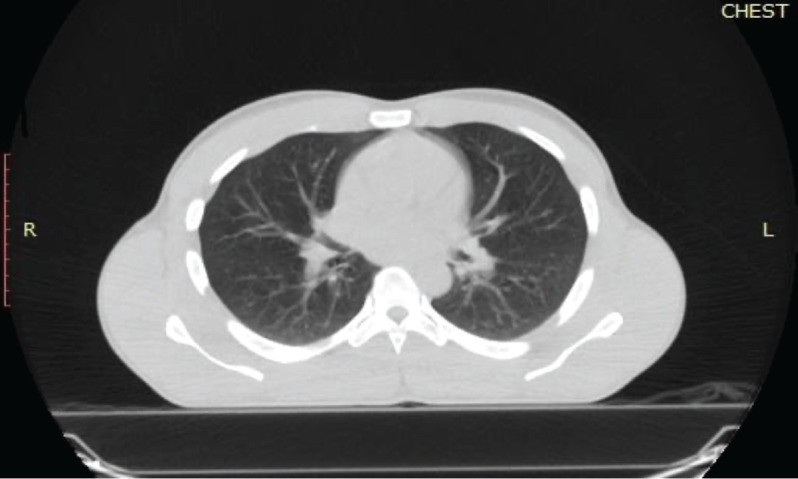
Reassessment chest CT scan of the patient. The lung window of a chest CT scan of the patient was done after 3 months of systemic therapy. It shows bilateral lung fields with no evidence of atelectasis or pulmonary nodules.
